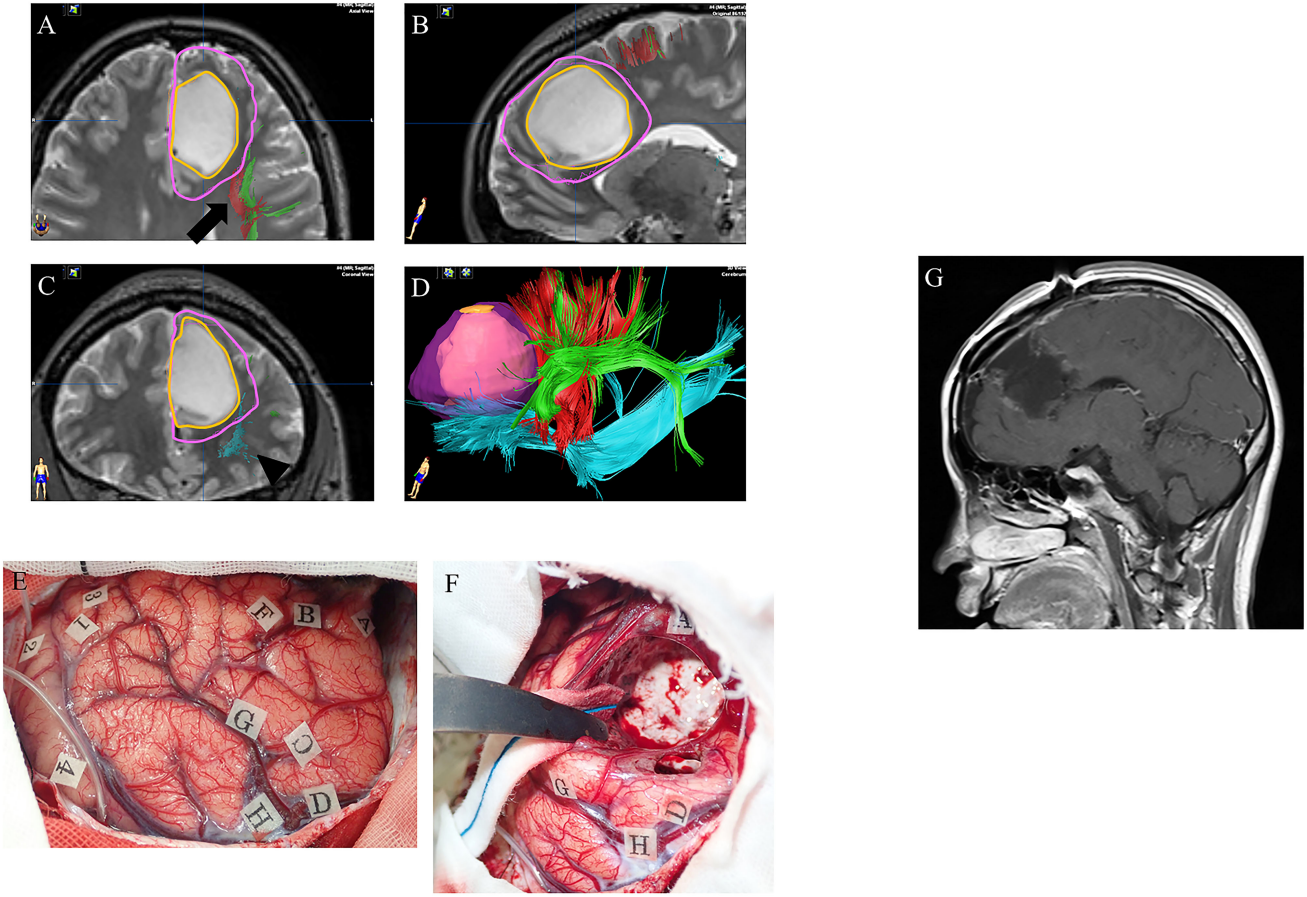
A case of left frontal lower-grade glioma in a 30-year-old right-handed female with no relevant medical history. Preoperative axial (A), sagittal (B), and coronal (C) T2-weighted MRI show a high intensity mass in the left frontal lobe. Preoperative three-dimensional tractography (D) shows the tumor itself (orange) and the planned resection area (violet; supratotal resection) surrounded by FAT (red; arrow in panel A), SLF (green; arrow in panel A) and IFOF (blue; arrowhead in panel C). Red, FAT; green, SLF; blue, IFOF; FAT, frontal aslant tract; SLF, superior longitudinal fasciculus; IFOF, fronto-occipital fasciculus. Intraoperative photograph prior to resection (E), showing letter tags that indicate tumor boundaries (A–D) and the planned resection area for supratotal resection (E–H). Stimulation over the precentral gyrus induced speech arrest (number tags: 2 and 4), as did stimulation over the opercular part of the inferior frontal gyrus (number tags: 1 and 3). (F) Intraoperative photograph obtained after supratotal resection. Stimulation over the IFOF induced semantic paraphasia (number tag: 31), and stimulation over the FAT induced speech arrest (number tags: 33, 34). FAT, frontal anterior tract; IFOF, fronto-occipital fasciculus. Postoperative sagittal T1-weighted MRI with gadolinium enhancement (G) showing supratotal resection with awake brain mapping.
